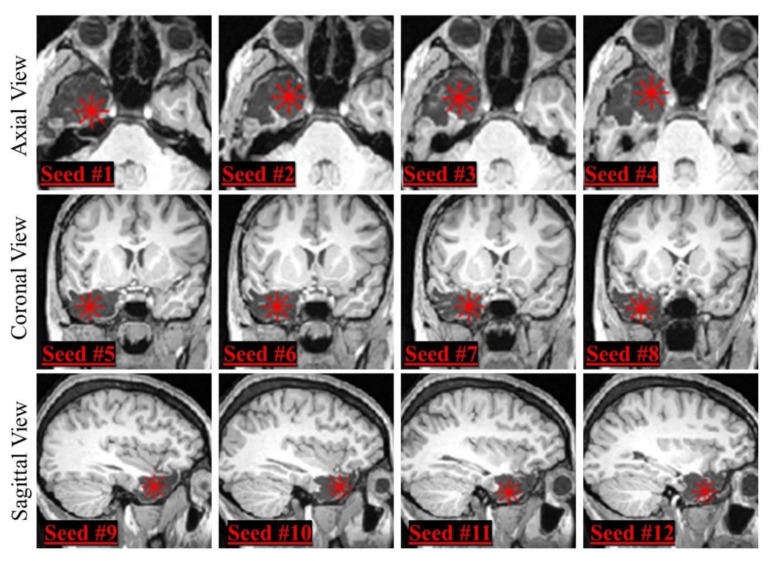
Seed positioning in different MRI views. We selected four seeds (indicated by red stars) from different slices from each MRI view (first row: axial view, second row: coronal view, third row: sagittal view), obtaining a total of 12 different seeds for each patient.
